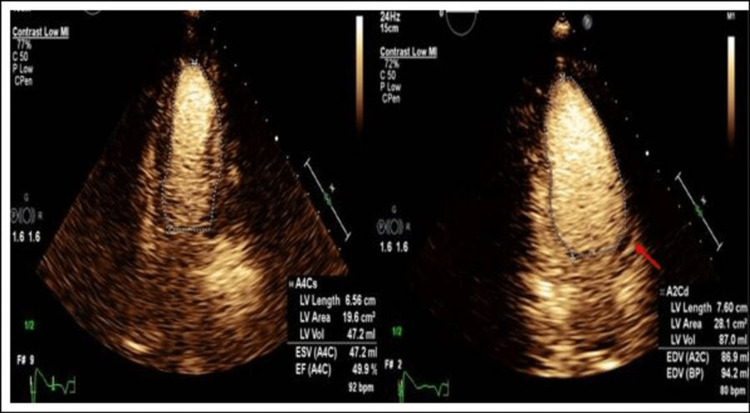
Follow-up echocardiogram with a significantly improved ejection fraction. Three days later, echocardiogram showed a remarkable improvement in overall wall motion abnormalities and an LVEF of 50%. Focal areas of akinesis involving the mid-anterolateral and mid-anterior segments (red arrow).
